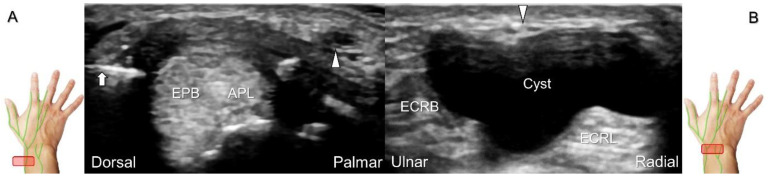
Sonographic imaging of the superficial radial nerve beside the first extensor compartment of the wrist (A), and a ganglion cyst over the second extensor compartment of the wrist (B). White arrowheads: superficial radial nerve; white arrow: needle. APL: abductor pollicis longus tendon; EPB: extensor pollicis brevis tendon; ECRL: extensor carpi radialis longus tendon; ECRB: extensor carpi radialis brevis tendon.
